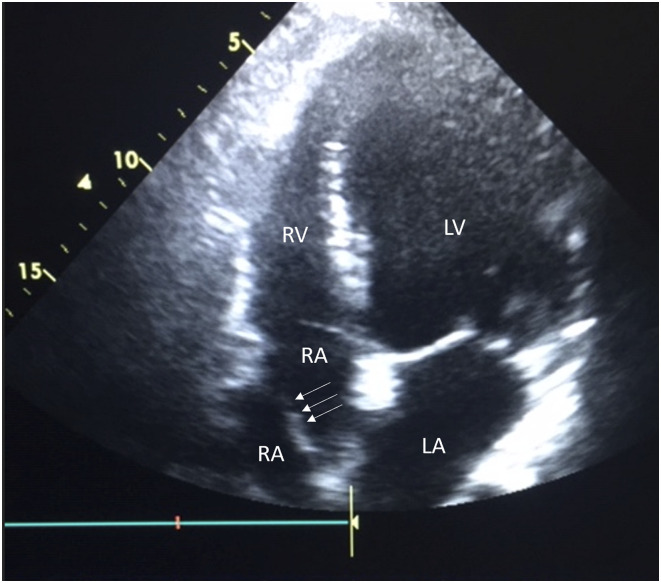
Transthoracic echocardiogram showing CTD. A membranous defect extending across the diameter of the right atrium and attaching to the atrial septum is visible.
